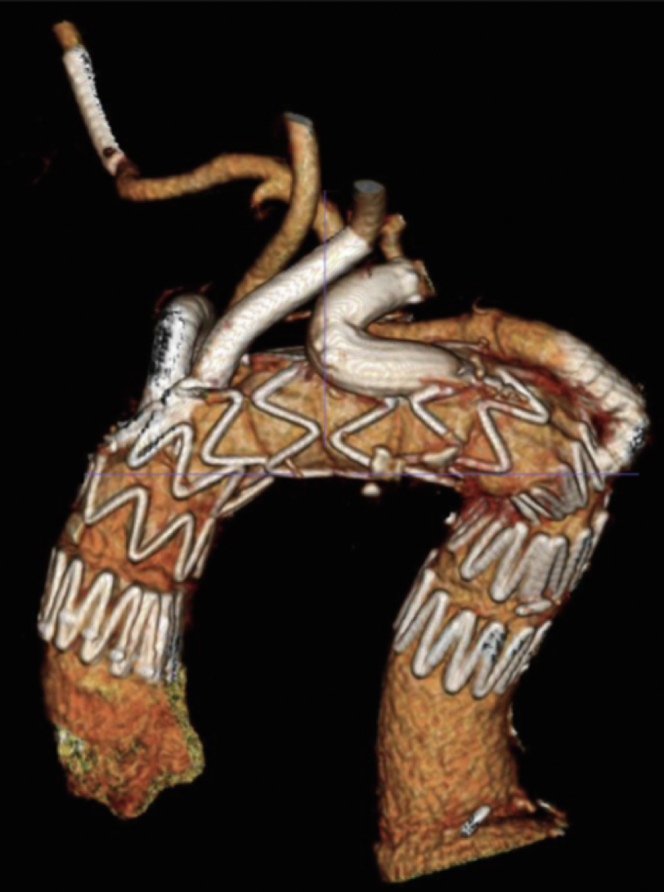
Postoperative 3-dimensional computed tomography angiography reconstruction of the 4-vessel aortic arch repair.
